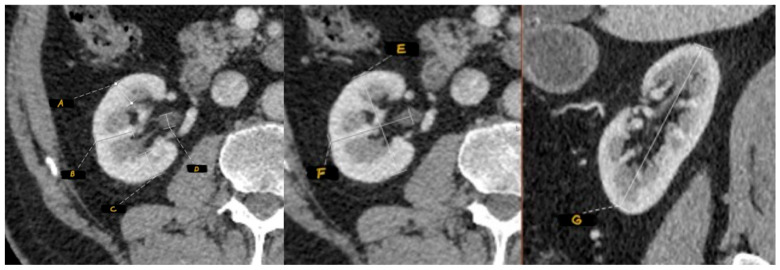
Measurements of the right renal pelvis AP diameter, average parenchymal thickness, and kidney volume on axial and sagittal CT imaging. A: Anterior parenchymal thickness (mm), B: lateral parenchymal thickness (mm), C: posterior parenchymal thickness (mm), D: renal pelvis AP diameter (mm), E: anteroposterior length of the kidney (mm), F: mediolateral width (mm), G: superoinferior height (mm).
